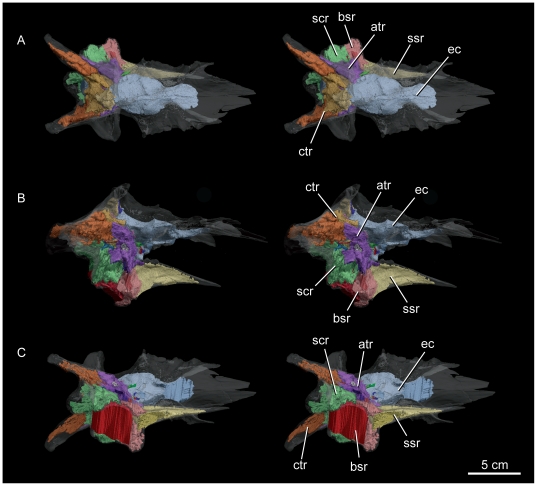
Stereopairs of the articulated braincase of *Alioramus altai* (IGM 100/1844). Dorsal (A), right lateral (B), and ventral (C) views. The images are reconstructed from the CT data with the bones rendered transparent to show the internal structures examined in this study. atr, anterior tympanic recess; bsr, basisphenoid recess; ctr, caudal tympanic recess; ec, endocranial cavity; scr, subcondylar recess; ssr, subsellar recess.
